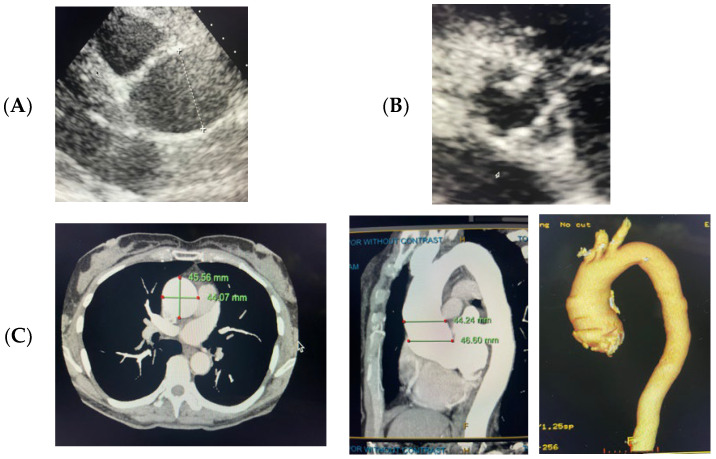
(A) Transthoracic echocardiographic PLAX view of aorta. (B) Transthoracic echocardiographic PSAX view of bicuspid aortic valve. (C) Aortic CTA with aortic measurements in 2D axial, 2D sagittal, and 3D reconstructions.
